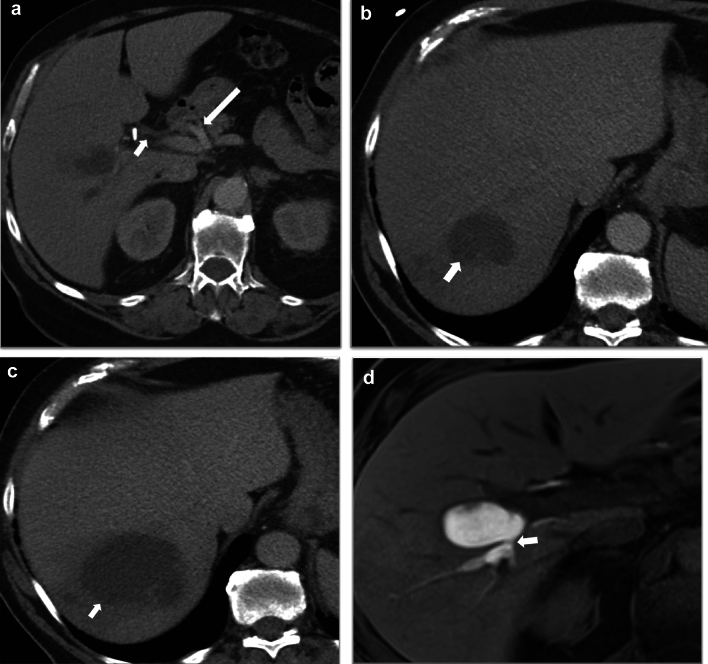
A 77-year-old male with right hepatic artery thrombosis post cholecystectomy leading to biliary ischemia in the right hepatic lobe and resulting in the formation of multiple bilomas. a Axial post-contrast CT demonstrating non-opacification of the right hepatic artery consistent with thrombosis (short arrow) and a surgical clip closely abutting it, likely representing inadvertent clipping of the artery and resultant thrombosis. The common hepatic artery (long arrow) and the left hepatic artery (not shown) were patent. b Axial CT post-contrast image shows a cystic lesion in the right hepatic lobe (arrow). Multiple such lesions were seen in the right lobe and were thought to be abscesses. c A follow-up CT performed after 1 month showed an increase in the interval size (arrow). Drain placement resulted in continuous bile drainage, confirming the diagnosis of bilomas. d) Axial post-Eovist MRI images show a biloma communicating with the biliary tree (arrow). No bilomas were seen in the left hepatic lobe. The cause for these multiple bilomas involving the right hepatic lobe was the right hepatic artery injury leading to biliary ischemia
